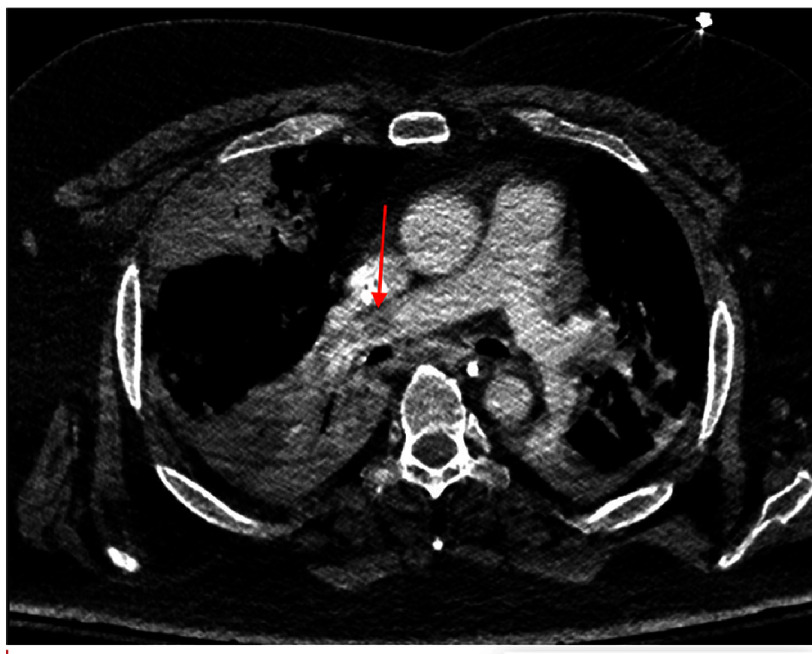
CTA of the chest showing that the previously seen saddle thrombus is not visualized, suggesting distal migration (arrow).
